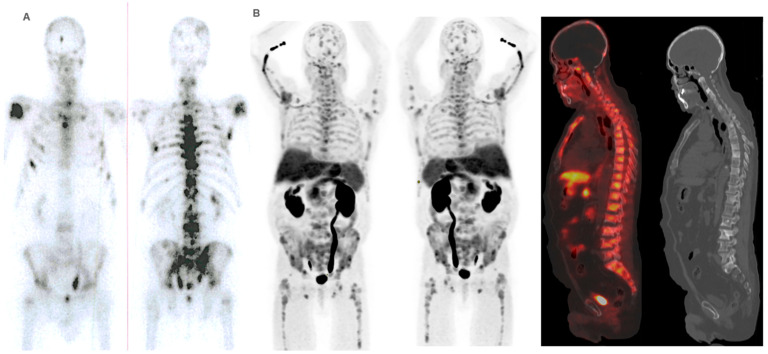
Patient ≠ 1. PSA and AP showed a steady increase after ^223^Ra initiation. Clinical deterioration was observed after the third ^223^Ra administration. Interim BS (A) and FCH PET/CT (B) show disease progression. The patient died 6 months after starting treatment with ^223^Ra.
